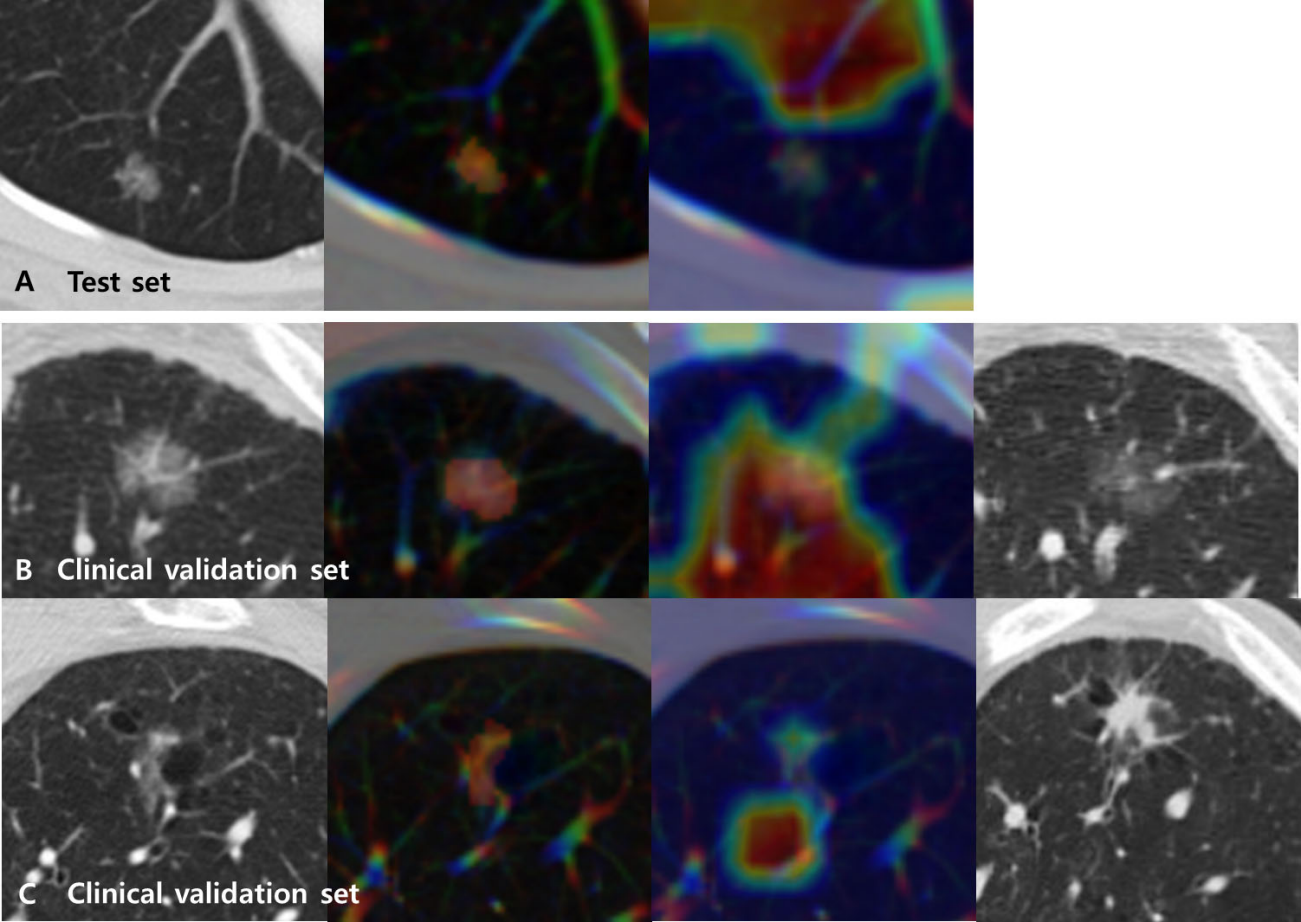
Representative CT images (first from the left) overlaid with regions of interest (ROIs) (second) and Grad-CAMs (third) for Multimodal EfficientNet-b1 for Lung (MENL) interpretation. (A) A *EGFR*-mutant correct case (probability 0.62) in the test set. (B) A *EGFR*-mutant (response) correct case (probability 0.69) in the clinical validation set. Compared to the baseline CT image (first), the last follow-up CT image after TKI (fourth) demonstrates a decrease in density. (C) A *EGFR*-wild type (non-response) correct case (probability 0.51) in the clinical validation set. Compared to the baseline CT image (first), the last follow-up CT image after TKI (fourth) demonstrates an increase in size and density. In all cases, the tumor and its proximal bronchovascular bundle are activated by the MENL.
